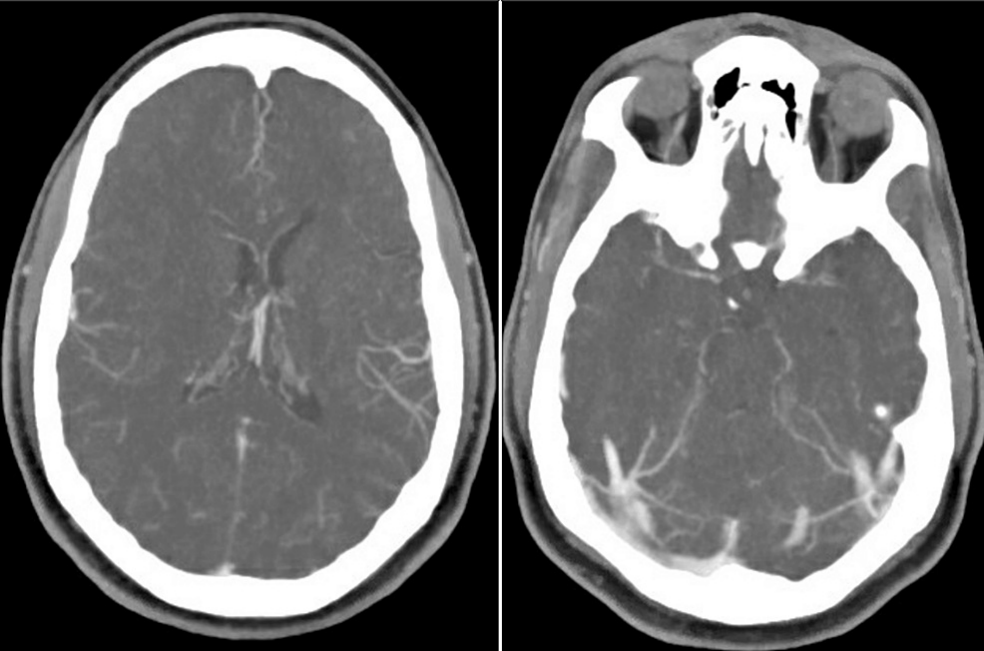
Axial CTA images showing numerous small caliber collateral vessels within the brain. CTA = Computed Tomography Angiography
